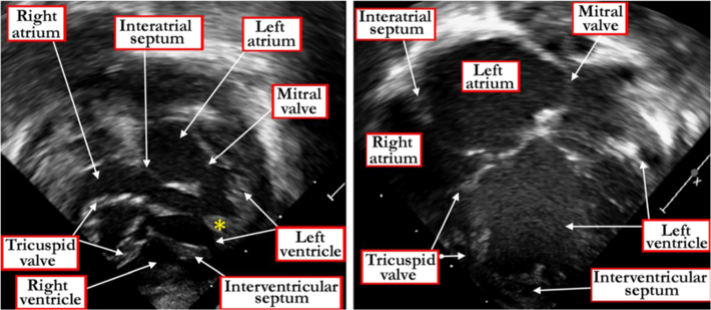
The panels show subcostal four chamber echocardiographic cuts revealing malalignment between the atrial and muscular ventricular septal structures in a heart with straddling and overriding of the tricuspid valve. Note the tendinous cords attached to a papillary muscle (yellow asterisk) within the left ventricle. The left hand panel shows the diastolic, and the right panel the systolic location of the overriding leaflets.
